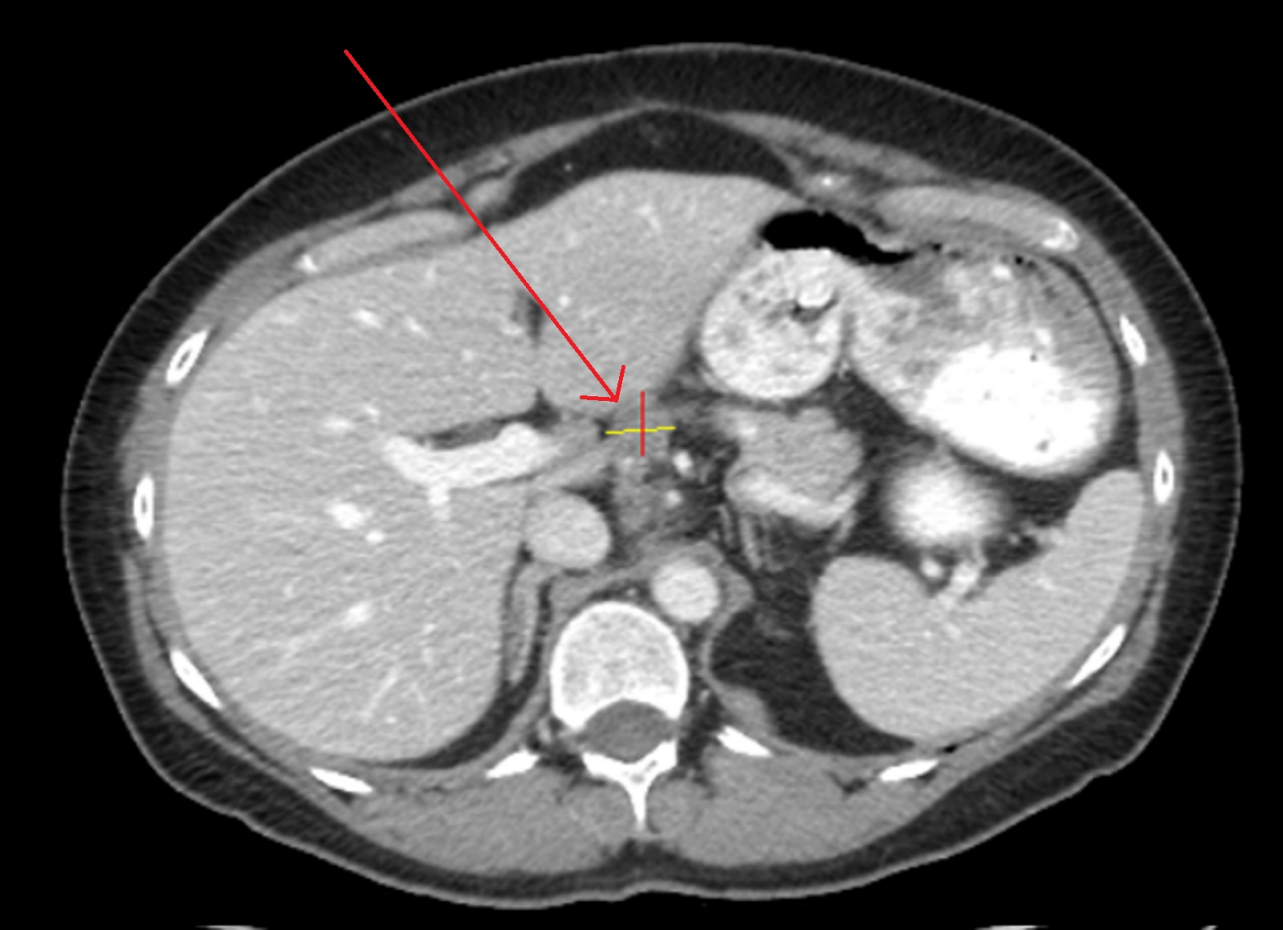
Abdominal CT of Patient 1 after completion of evofosfamide regimen, with stability in target lesion at 1.8 x 2.0 cm, with CA-125 107 U/ml
